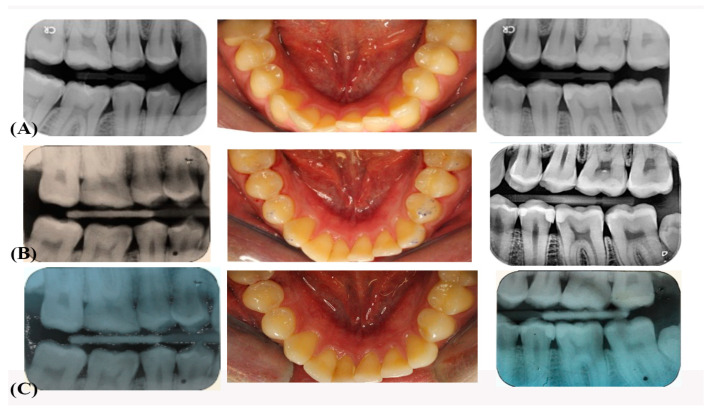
(A) Pre-operative clinical photograph and bitewings radiographs on teeth 45 and 35 occluso distally (B) Clinical photograph and bitewings radiographs on teeth 45 and 35 occluso distally at T0 (C) at 2 weeks.
